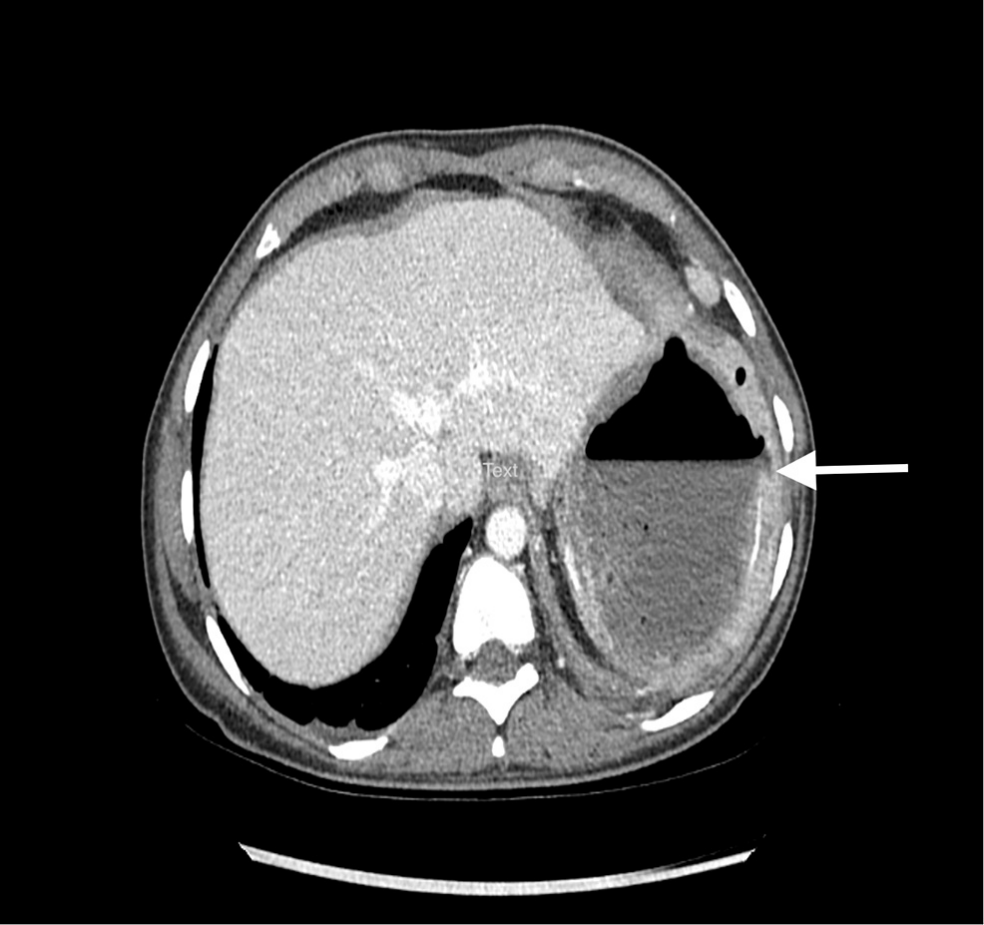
CT scan of the abdomen showing a splenic abscess with an air-fluid level (white arrow).
